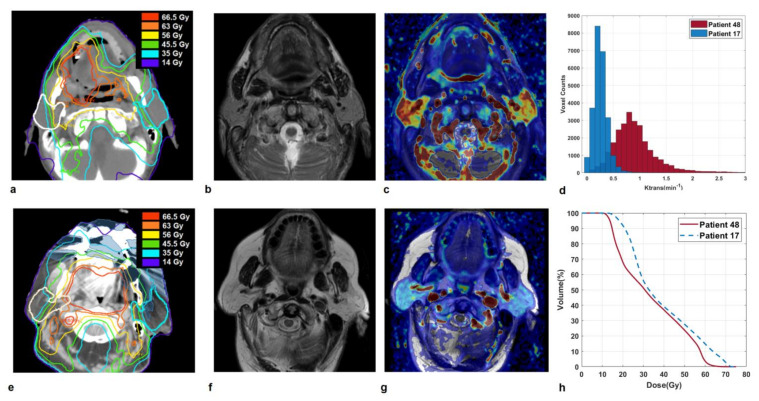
Sections of parotid glands in a 68-year-old man affected by a tonsil carcinoma (Patient 48) on axial CT image with overlaid isodose levels and parotid contours (a); the corresponding axial T2-weighted image (b) and map of K^trans^ (c) which indicates highly vascularized glands. Analogously, sections of parotid glands in a 75-year-old woman affected by a soft palate carcinoma (Patient 17) on axial CT image with overlaid isodose levels and parotid contours (e); the corresponding axial T2-weighted image (f) and map of K^trans^ (g) which shows moderately perfused glands. K^trans^ histograms (d) and dose-volume histograms (h) of both parotids, for Patient 48 and 17 in comparison. Patient 48, who developed grade 1 XER_12_, had much more vascularized parotids glands than Patient 17, who experienced grade 2 XER_12_ (median K^trans^ = 0.93 versus 0.19 min^−1^). He received little lower doses to parotids (D_mean_/V65 = 33.3 Gy/0.4% versus 38.1 Gy/8.5%) but showed much lower in-treatment XQ scores, compared to patient 17 (XQ-Int_mid_ = 33.2 versus 82.9, respectively).
